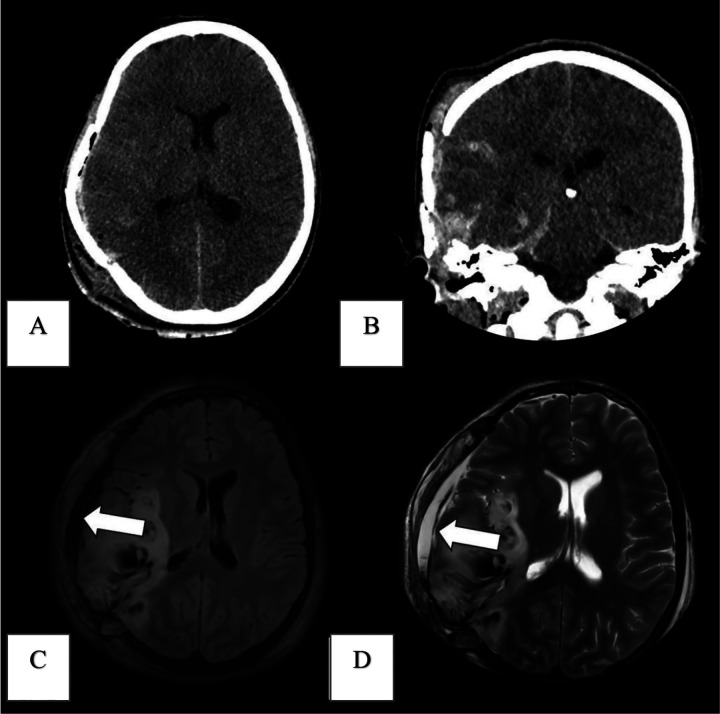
Computed tomograms of the patient’s brain at admission, A (axial section), B (frontal section). MRI of the patient after DC. C (axial section T1 mode), D (axial section T2 mode). The arrow shows the development of an acute subdural hematoma in the early postoperative period.
